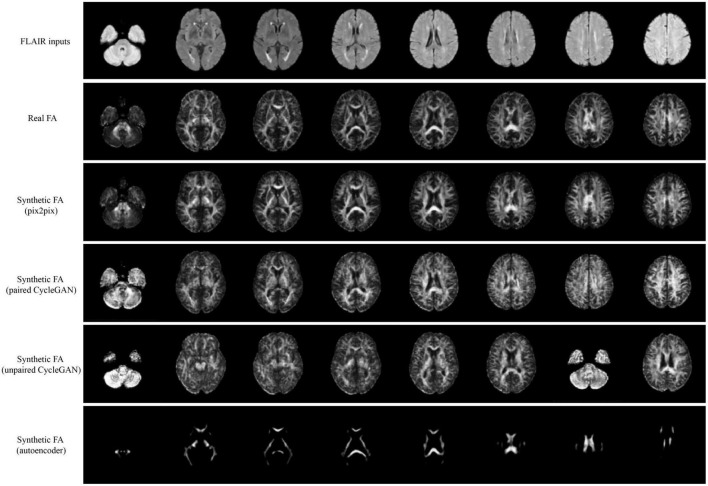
Samples of FLAIR inputs (top row), real FA (second row) and generated FA slices from each model. For paired models, the images shown are different slices from the same patient volume. From left to right, randomly sampled lower to upper slices (12 to 35) are shown.
